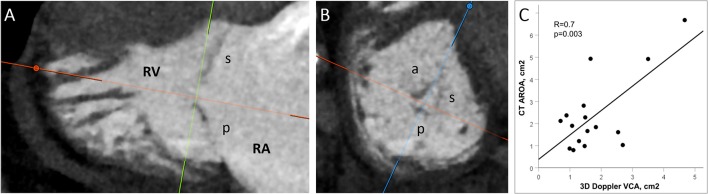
Multiplanar reconstruction of the anatomic regurgitant orifice area (AROA) on MSCT images. (A) The reformation planes are adjusted at the leaflet tips during systole (20–40%). (B) The regurgitant orifice is delineated on the short axis. The AROA in this case is 0.7 cm^2^. (C) Correlation between TEE color-Doppler 3D vena contracta area (VCA) and MSCT AROA obtained with multiplanar reconstruction.
